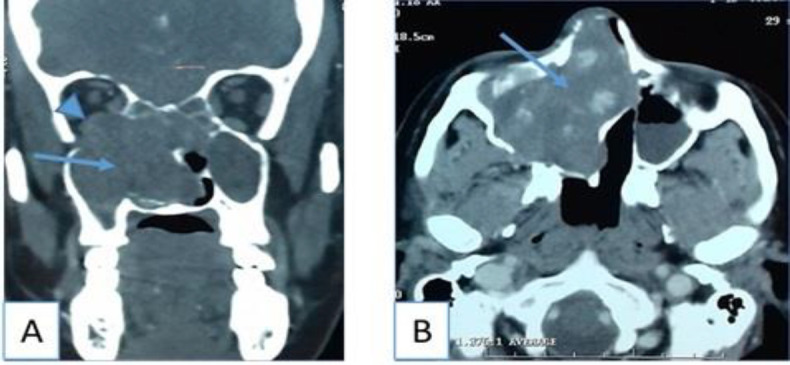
CT of the paranasal sinuses: (A) nonenhanced coronal section shows heterogenous soft-tissue mass (arrow) in the right maxillary sinus, the ethmoid and the nasal cavity with destruction of the medial wall of the sinus and the nasal septum, destruction of the orbital floor and extension of the mass into the peri-orbita (arrow head). (B) enhanced axial section shows nonhomogeneous enhancement
